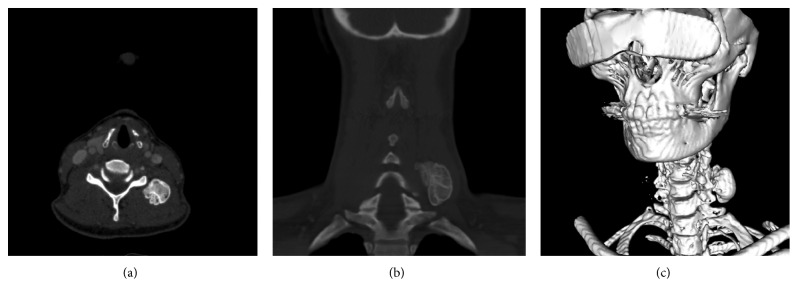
Preoperative computertomography scan: axial (a), coronary (b), and 3D-reconstruction (c). The CT scan shows a calcificated mass without contact to the skeleton.
